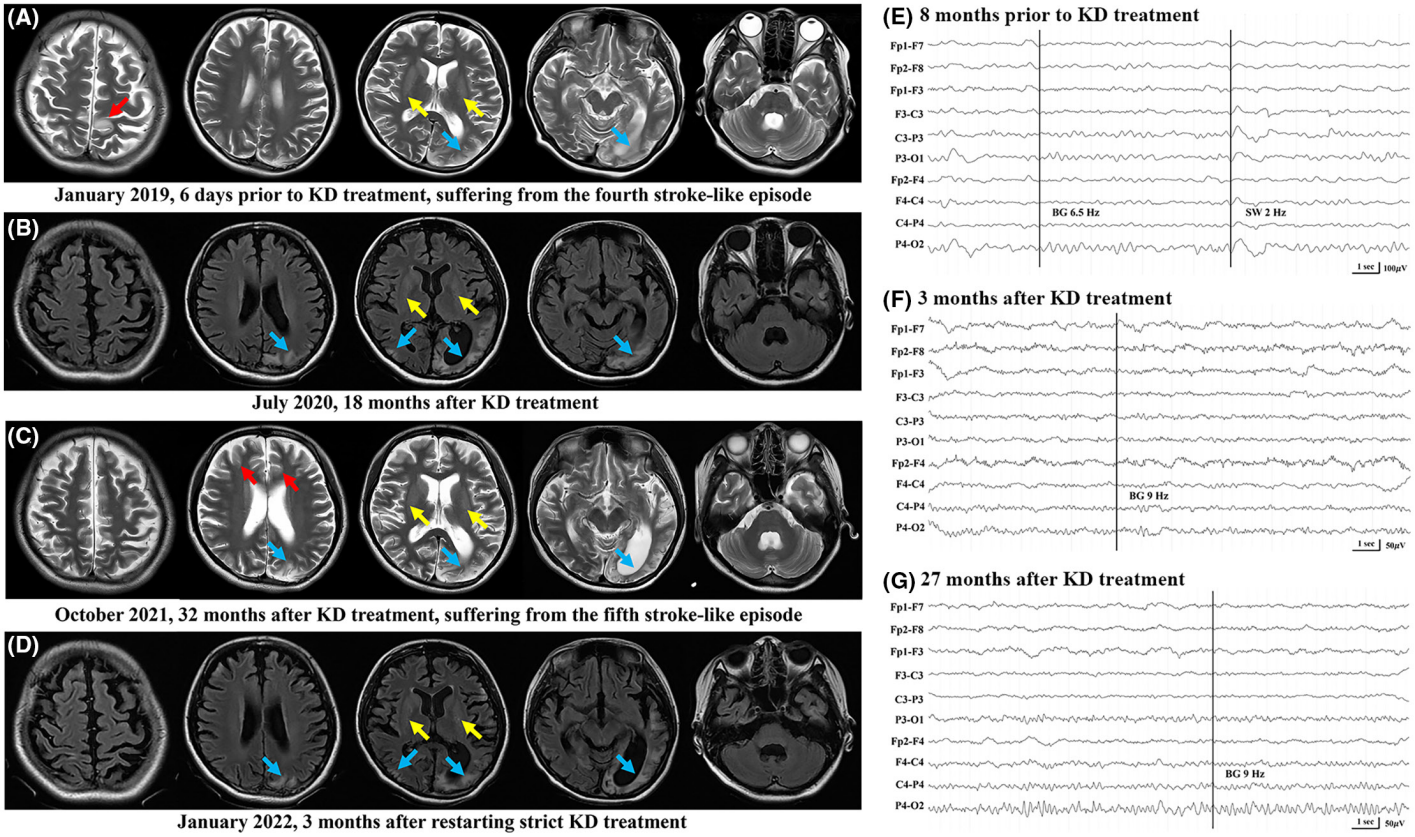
Chronological MRI findings and EEG examinations. (A) Six days prior to KD treatment, MRI (T2 images) showed a new lesion in the right parietal lobe, obsolete lesions in the bilateral posterior quadrants (more severe on the left) and mild cortical atrophy and bilateral basal ganglia calcification. (B) Eighteen months after KD treatment, T2‐FLAIR images showed no apparent change of the cortical volume. (C) On her fifth stroke‐like episode caused by non‐compliance with KD treatment, T2 images showed new lesions in the bilateral frontal lobes and the right parietal lobe were detected. (D) Three months after restarting strict KD treatment, the new responsible lesions resolved (T2‐FLAIR images). (A–D) Bilateral basal ganglia calcification (yellow arrows) was consistently observed. New lesions, red arrows; obsolete lesions, blue arrows. (E) Eight months prior to KD treatment, EEG recording showed a posterior background of 6–7 Hz and intermittent diffused slow activity of 2–4 Hz, which suggested a moderate encephalopathy. (F) Three months after KD treatment the posterior background was normalized (8–9 Hz) and maintained thereafter (G). Meanwhile, diffuse slowing and a normal posterior background suggested a mild encephalopathy. EEG, electroencephalogram; FLAIR, fluid attenuated inversion recovery; KD, ketogenic diet; MRI, magnetic resonance imaging.
